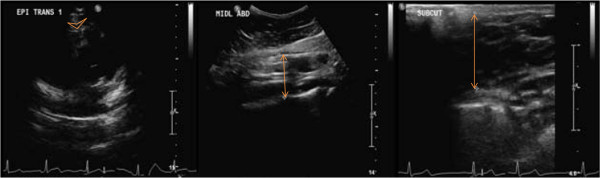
Ultrasonographic epicardial (left), intraabominal visceral (middle) and abdominal subcutaneous adipose tissue (right) in B-mode ultrasonography.
